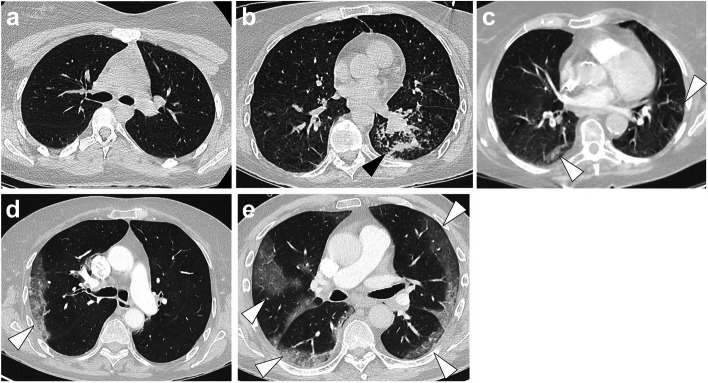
French ‘*Société Française de Radiologie*’ and ‘*Société Française d’Imagerie Thoracique*’ adapted classifications for the radiological findings in the setting of a suspicion of coronavirus disease 2019 (COVID-19), or SFR-SIT diagnostic score, categorised as the following: a I: no pathologic findings; b II: non-SARS-CoV-2 infections (herein, bacterial bronchopneumonitis, black arrowheads); c III: indeterminate (herein, subtle subpleural condensations, white arrowhead); d IV: pathologic findings compatible with COVID-19 (herein, a unique subpleural area of ground glass opacity, white arrowhead); e V: pathologic findings typical for COVID-19 lung disease (i.e. multiple ground glass opacities seen in ≥ 2 lobes, with a subpleural predominant locations, possibly associated with band-like condensations and reversible fibrosis). All CT images are in axial plane and lung kernel
